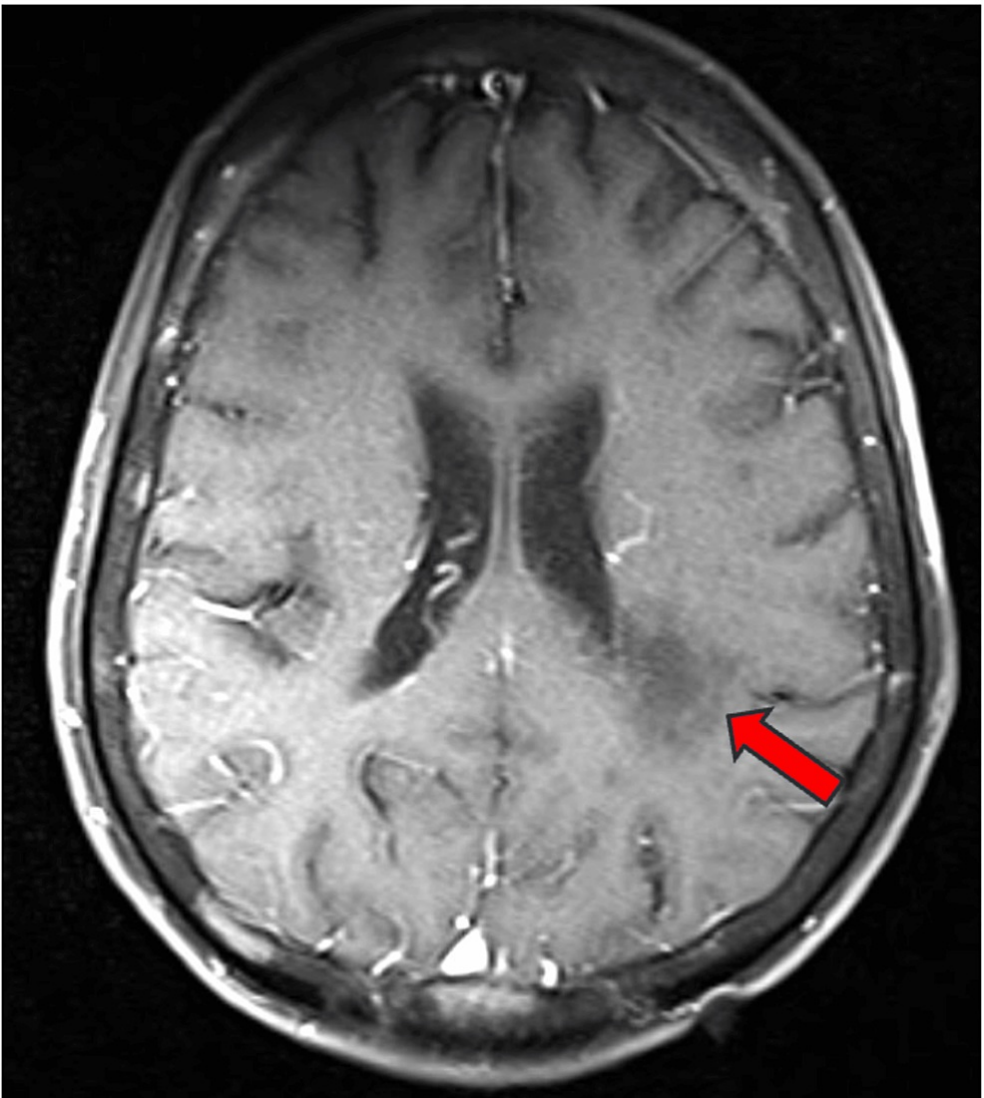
MRI brain (P+C) T1 post-contrast axial weighted image showing no enhancement of the lesion in the left peritrigonal region is shown by the red arrow. MRI brain (P+C): Magnetic resonance imaging brain plain and contrast
